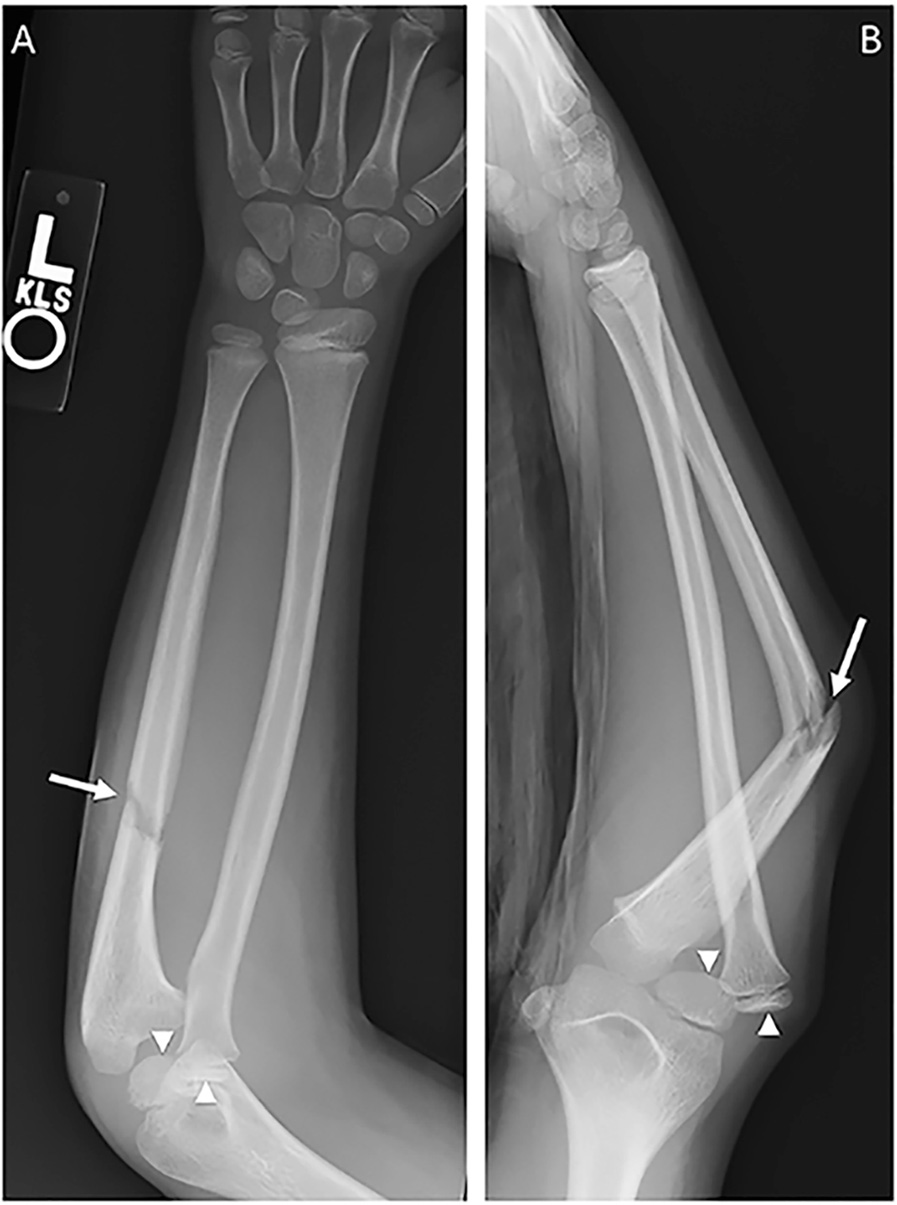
Monteggia fracture. PA (a) and lateral (b) radiographs of the forearm demonstrate angulated fracture of the proximal ulna (*arrow*) and dislocation of the radial head from the capitellum (*arrowheads* on both the radial head and capitellum)
